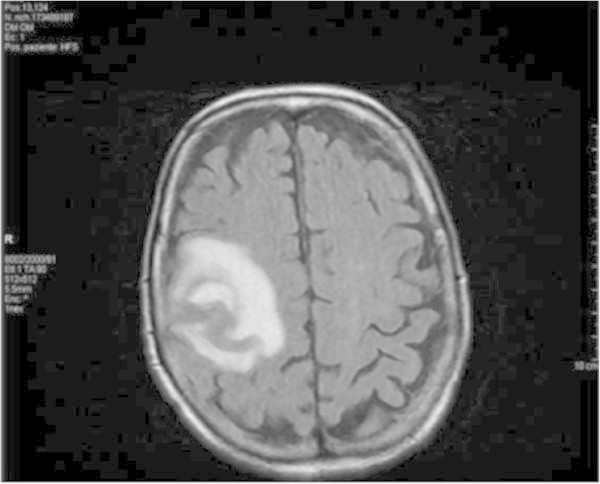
MR imaging showing a proliferative lesion on right fronto-rolandic region.
